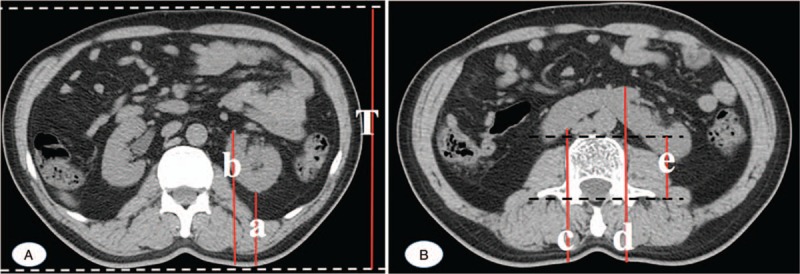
(A) CT scan showing skin to anterior and posterior renal surfaces at the level of the renal hilum. Renal depth was determined by averaging the anterior and posterior depths at the renal hilum: renal depth (cm) = (*a* + *b*)/2; *T* is total thickness of the body at the level of the kidneys. (B) Isthmus depth was determined by averaging the highest and lowest points on both sides of the isthmus vertebrae at the maximum cross sectional level of isthmus, isthmus depth (cm) = (*c* + *d*)/2; vertebral thickness (*e*) was determined from the anterior edge of the vertebral body to the transverse process of the vertebral body. Isthmus soft tissue depth = (*c* + *d*)/2 – *e*.
